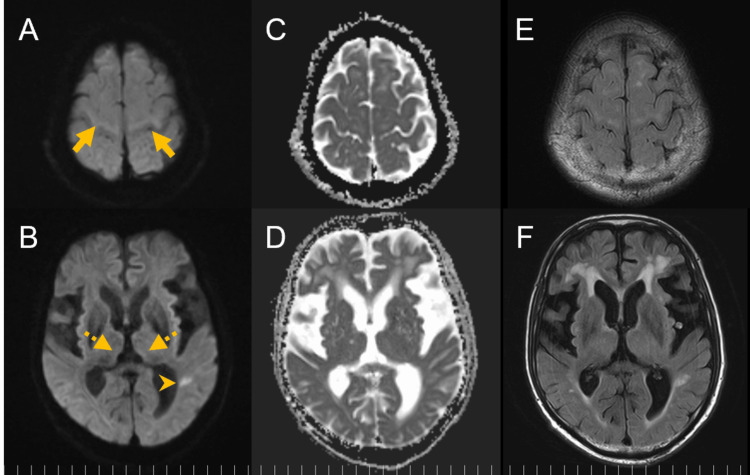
Brain MRI 32 days after the onset of altered mental status, by which time the patient’s mental status had recovered (A, C, E) The cortical ribboning in both frontal cortices (arrows) has almost completely resolved. DWI and ADC are normalized, and only a slight high signal is observed on FLAIR. (B, D, F) Abnormalities in the medial thalami bilaterally (dashed arrows) are no longer prominent. The left parietal subcortical lesion (arrowhead) still shows a high signal on DWI and FLAIR, with normal ADC, suggesting that it represents a time-dependent change in cerebral ischemia. ADC, apparent diffusion coefficient; DWI, diffusion-weighted imaging; FLAIR, fluid-attenuated inversion recovery
